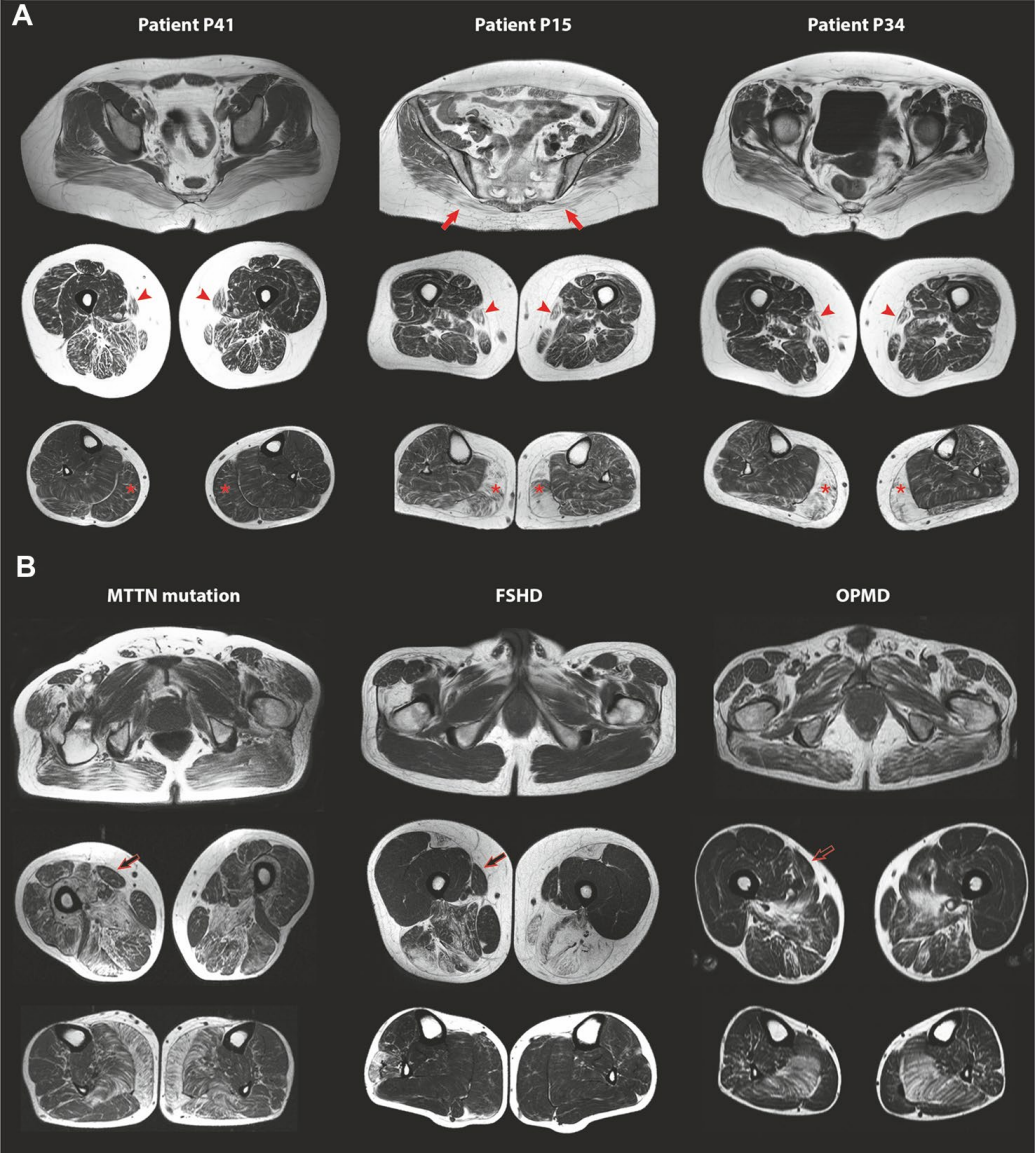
Muscle MRI pattern of the lower limbs in TK2d patients compared to other myopathies. A Muscle MRI from three TK2d patients with different disease duration and weakness severity showed that the most affected muscles were the *gluteus maximus* (arrows), the *sartorius* (arrowheads), and the *gastrocnemius medialis* muscles (asterisks). B Muscle MRI from patients with other mitochondrial myopathies as the one linked to point mutation in the mitochondrial gene MTTN, or other myopathies with similar clinical characteristics as FSHD or OPMD, showed a very different pattern of muscle involvement, highlighting the full preservation of the *sartorius* muscles (open arrows), even when other muscles are severely degenerated
